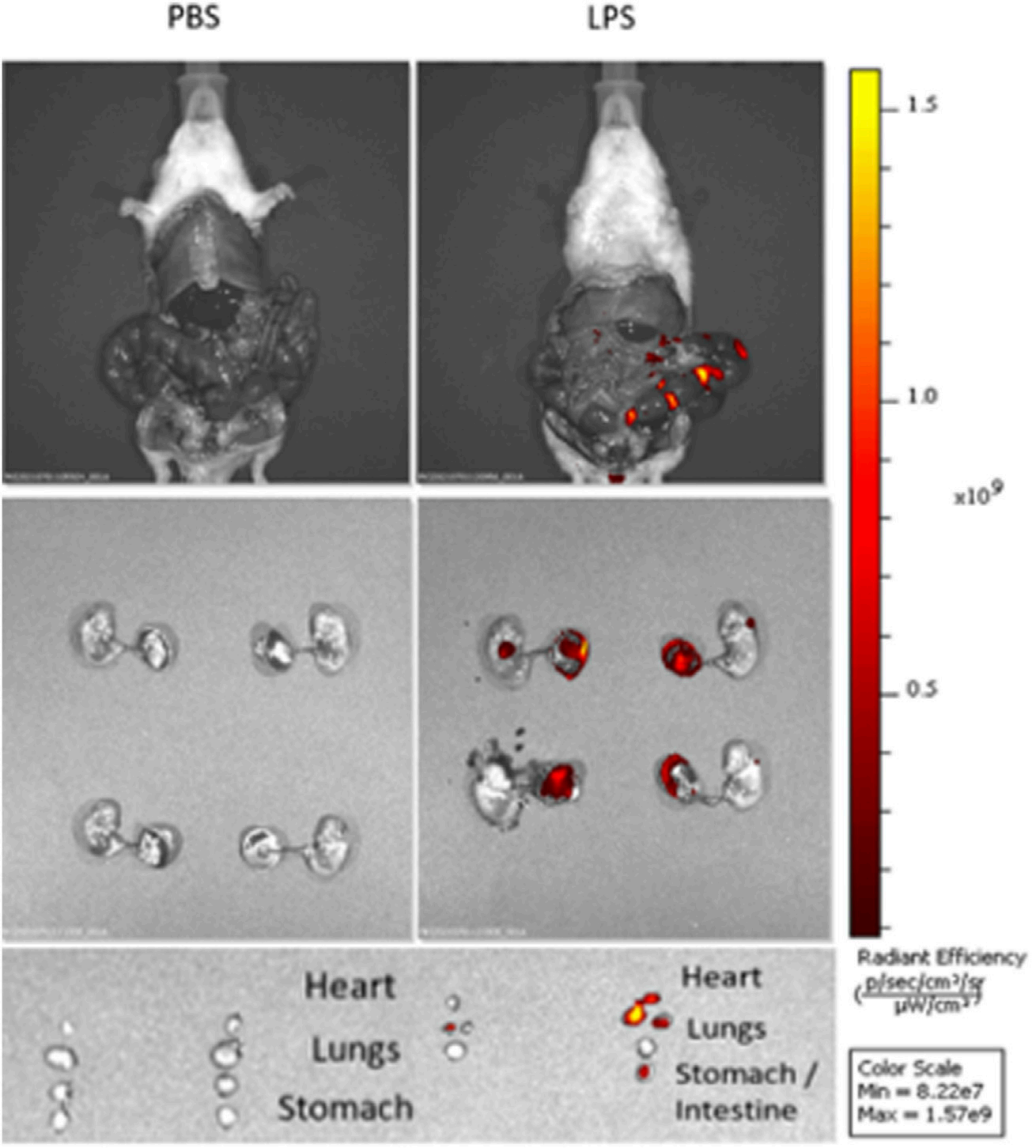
Intra-amniotic LPS localization and c-Myc expression in placenta. Distribution of LPS after intra-amniotic injections visualized by *in vivo* fluorescent imaging. Ultrasound-guided injections of LPS localized to amniotic cavity, fetal lungs and gastrointestinal tract.
